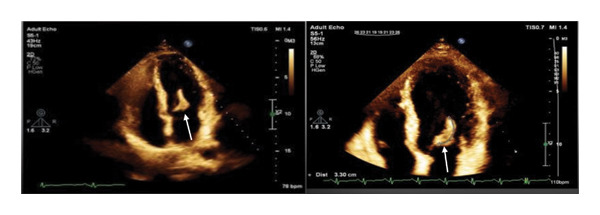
Apical four‐chamber view of transthoracic echocardiography. White arrows indicate an elongated, large, hypermobile mass with myocardial density measuring 44 by 11 mm, attached to the atrial side of the A3 scallop.
